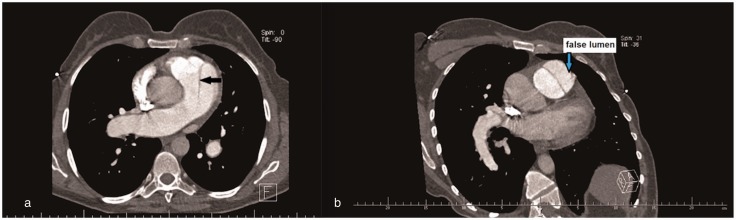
(a) CT scan showing dilated central pulmonary arteries with presence of a dissection flap in the main pulmonary artery (black arrow). (b) CT scan showing a linear dissection of the main pulmonary trunk with false lumen (blue arrow).
